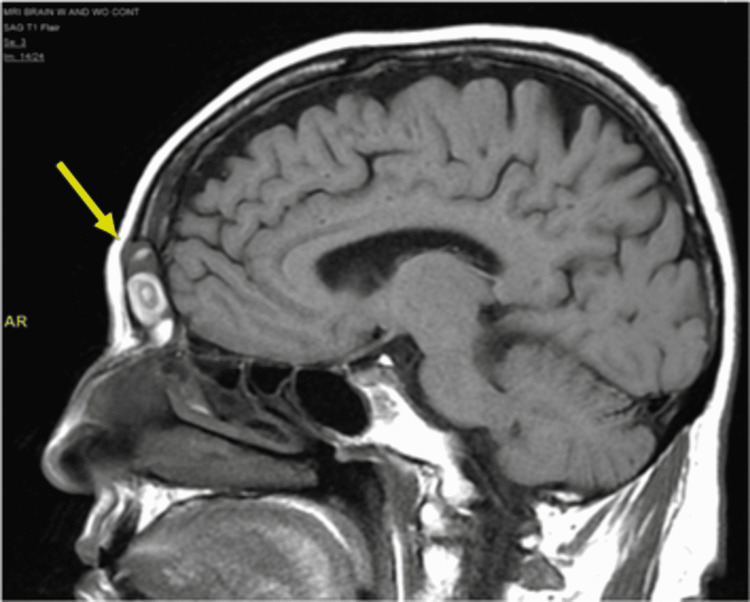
Brain MRI T1/FLAIR Complete opacification of the frontal sinus with disruption of the anterior cortex of the frontal sinus and protuberance of the opacification within the frontal sinus into the subgaleal soft tissues (arrow). No evidence of secondary intracranial involvement.
